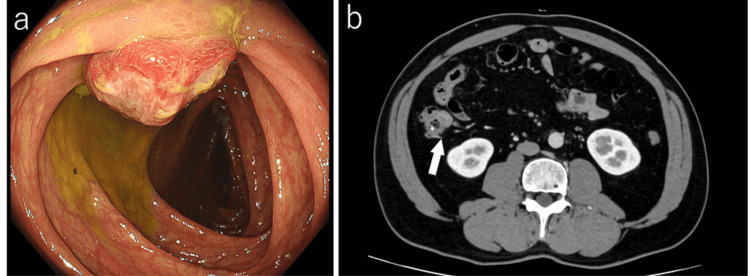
Colonoscopy and contrast-enhanced abdominal CT findings (a) Colonoscopy revealed a 20 mm elevated lesion with central ulceration in the ascending colon. (b) On contrast-enhanced abdominal CT, the clip placed near the tumor site was identified; however, the tumor itself was not clearly visualized. No liver metastases or ascites were observed.
